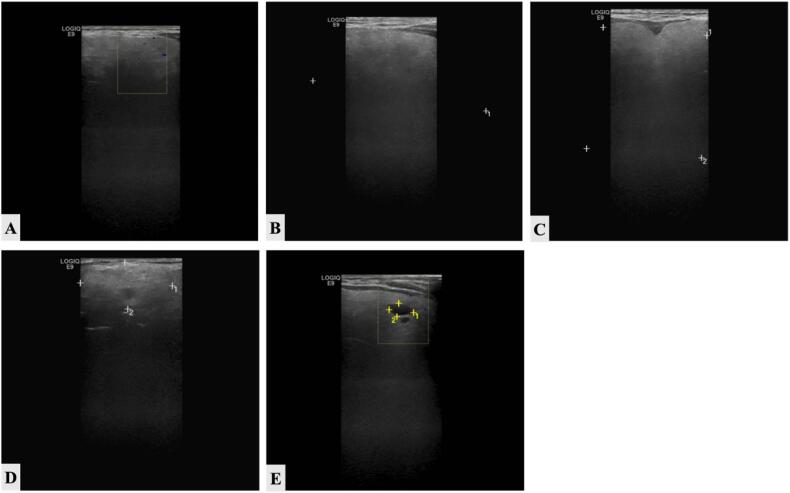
The ultrasound images of the thyroid gland. A, B, C, D, and E showed the ultrasound findings.
